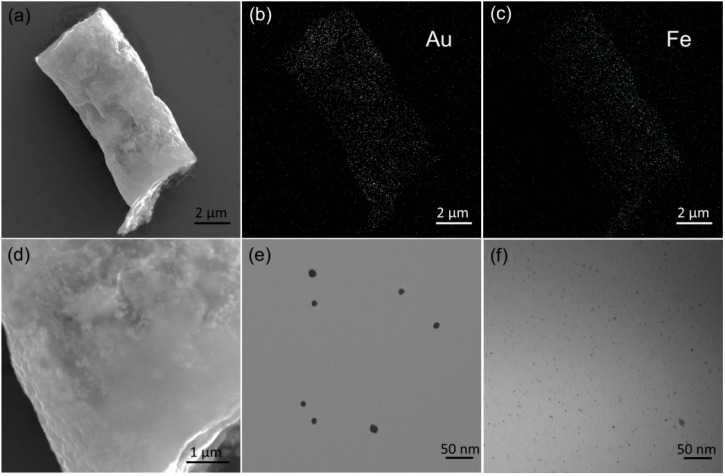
The characterization of the micro-tube. (a) SEM image of a single micro-tube; (b) The corresponding energy-dispersive X-ray (EDX) mapping images of (a) for Au distribution; (c) The corresponding EDX mapping images of (a) for Fe distribution; (d) Enlarged SEM image of the micro-tube; (e) TEM images of Fe_3_O_4_ NPs; (f) TEM images of Au NPs.
